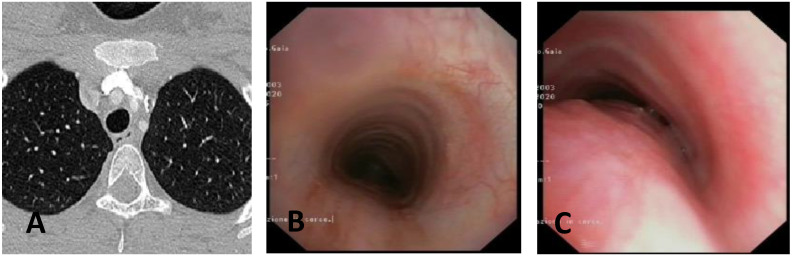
(A) Chest CT with CM: Well-preserved tracheal lumen even at the junction with the IA. Figure (B) SVBS: Persistent reduction in the lumen by 25–30% between the middle and distal third of the trachea, with no more visible transmitted pulsations and granulomas. (C) DVBS: Marked protrusion of the pars membranacea with abdominal straining, occluding the lumen by about 80–90%. Abbreviations. CT: Computed Tomography; CM: Contrast Medium; OAA: Open Anterior Aortopexy; IA: Innominate Artery; SVBS: Static Videobronchoscopy; DVBS: Dynamic Videobronchoscopy.
